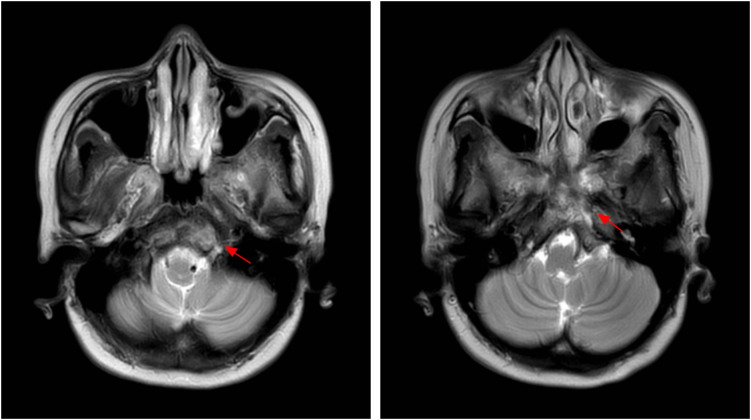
Pre-radiotherapy head MRI (T2W-MV sequence): Axial images demonstrate a well-defined hyperintense lesion involving the clivus (red arrows). The lesion measures approximately 3.3 cm in greatest dimension. No intracranial extension is observed. These findings are consistent with metastatic disease.
